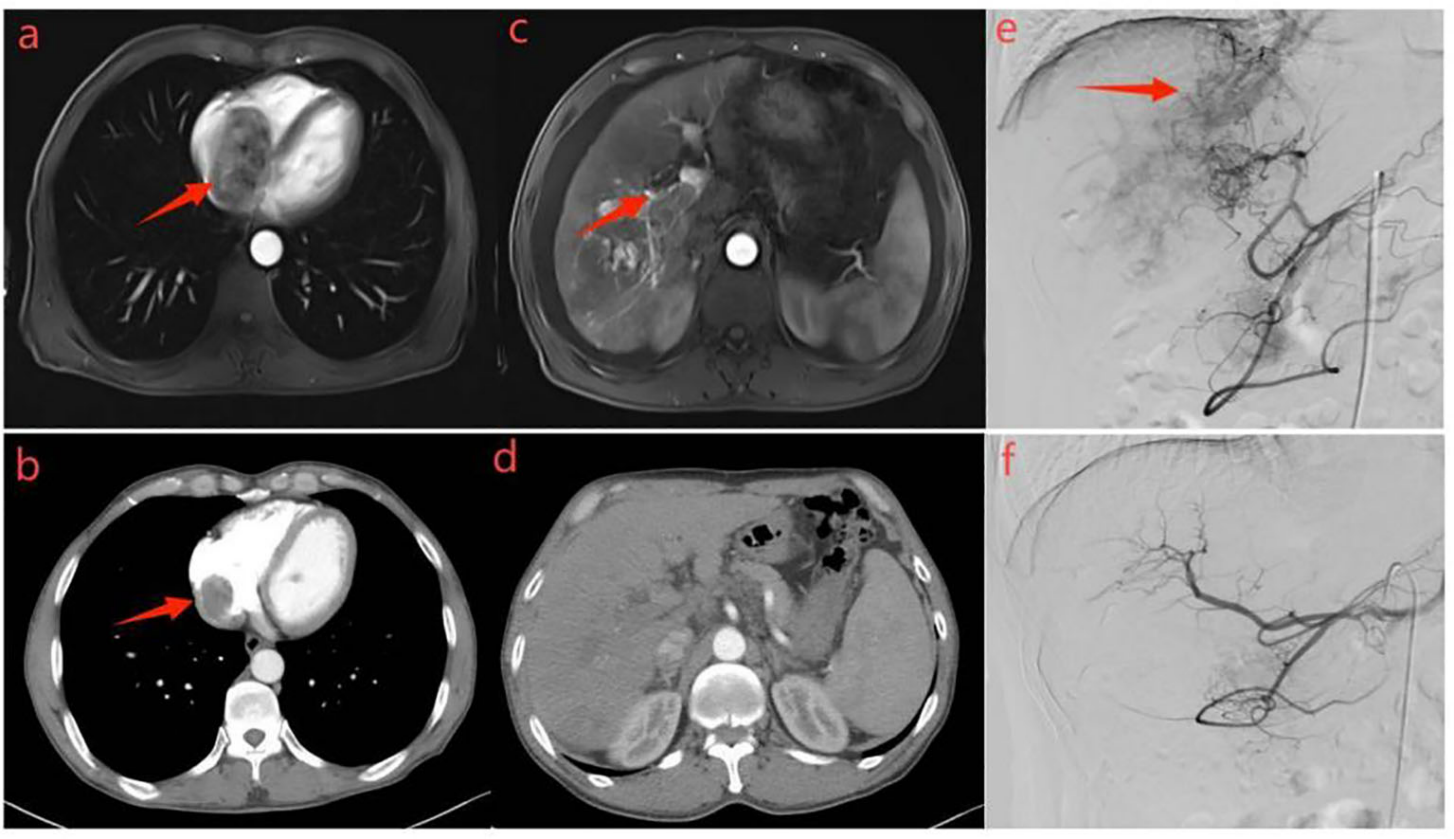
Representative radiographical images of a patient who achieved AVS disappearance after treatment. Images of man aged 65 years with HCC complicated by right atrial tumor thrombus and severe AVS after receiving triple therapy. Axial contrast-enhanced MRI images in the arterial phase (a) and portal phase (c) before the initiation of treatment. MRI images taken during the arterial phase (a) displayed early enhancement of tumor and right atrial cavity filling defect (indicated by a black arrow), representing AVS. Portal venous phase (c) showed filling defects in the main trunk and left branch of the portal vein, representing tumor thrombi in the portal vein (indicated by a black arrow).Hepatic angiography (e) revealed severe AVS and right atrial tumor thrombus (indicated by a black arrow). Subsequent axial contrast-enhanced CT images in the arterial (b) and portal (d) phase were taken after two cycles of triple therapy, showing no apparent APS and regression of right atrial tumor thrombus. Hepatic angiography in image (f) confirmed the absence of AVS.
